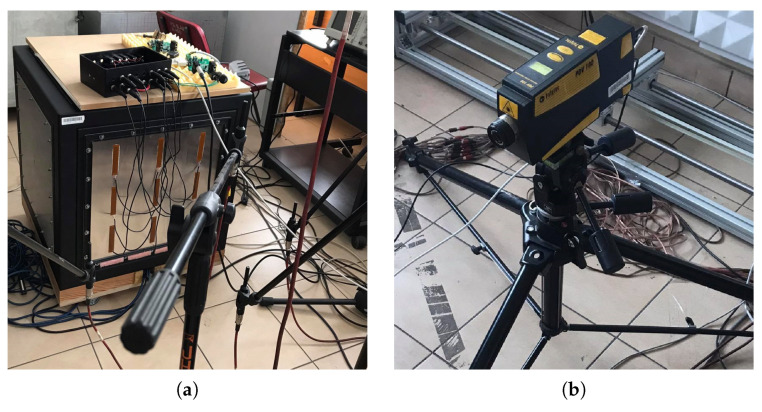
Pictures of the equipment used in the experiments: casing with MFC elements (a), laser Doppler Vibrometer (b).
